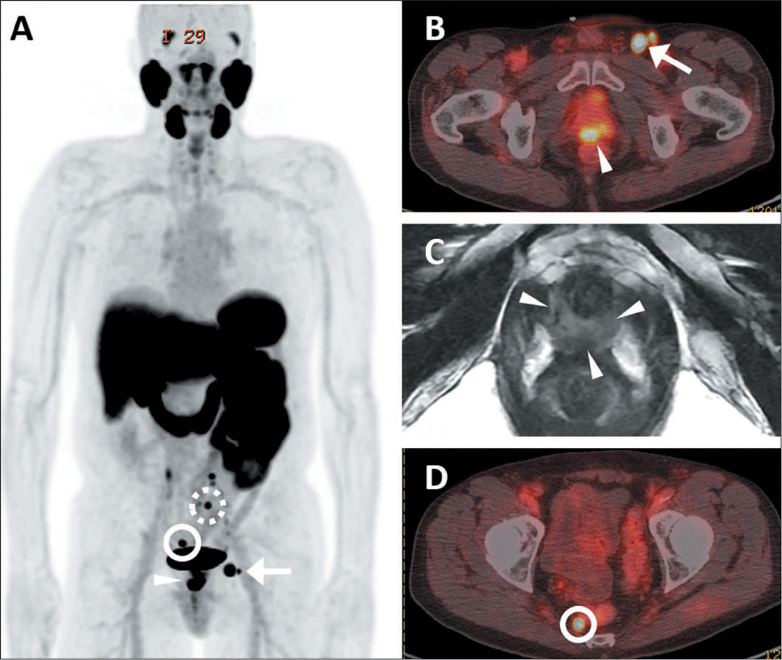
PSMA PET/CT and prostate MRI of a 66-year-old man with newly diagnosed ISUP grade
